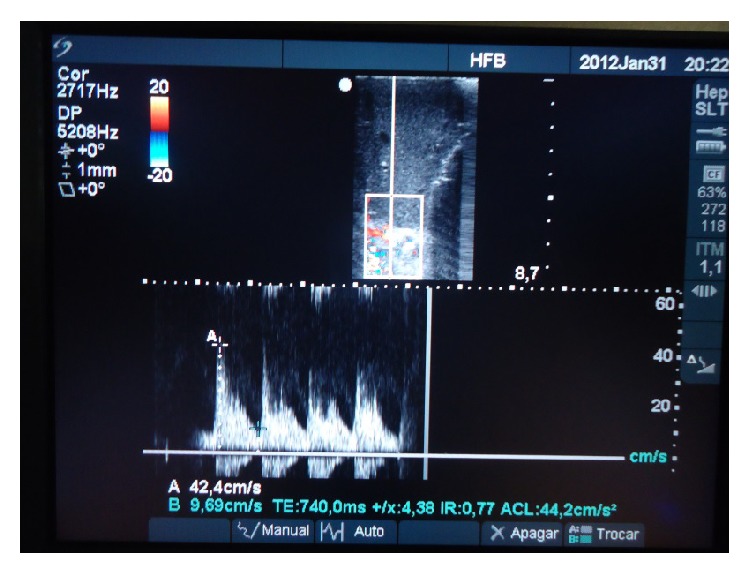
Intraoperative Doppler exam confirming intrahepatic arterial flow.
